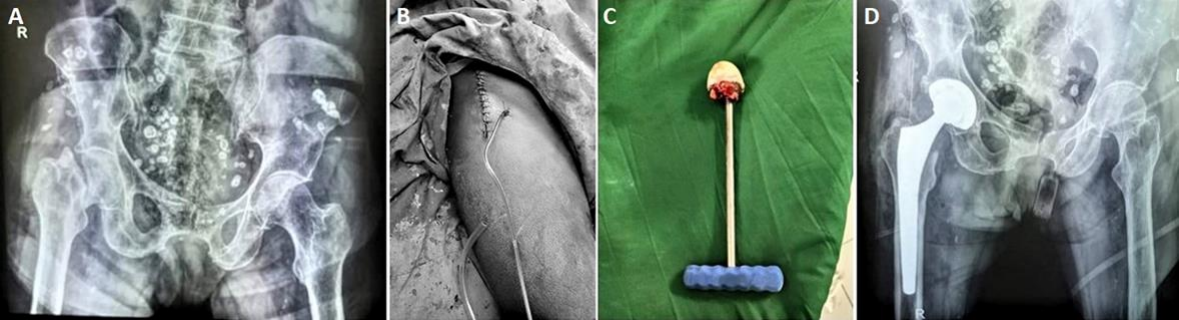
total hip arthroplasty for a neglected femoral neck fracture: A) preoperative X-ray; B) surgical wound and tubular drain; C) femoral head; D) postoperative X-ray, Maroua Military Hospital, Cameroon, 2024
